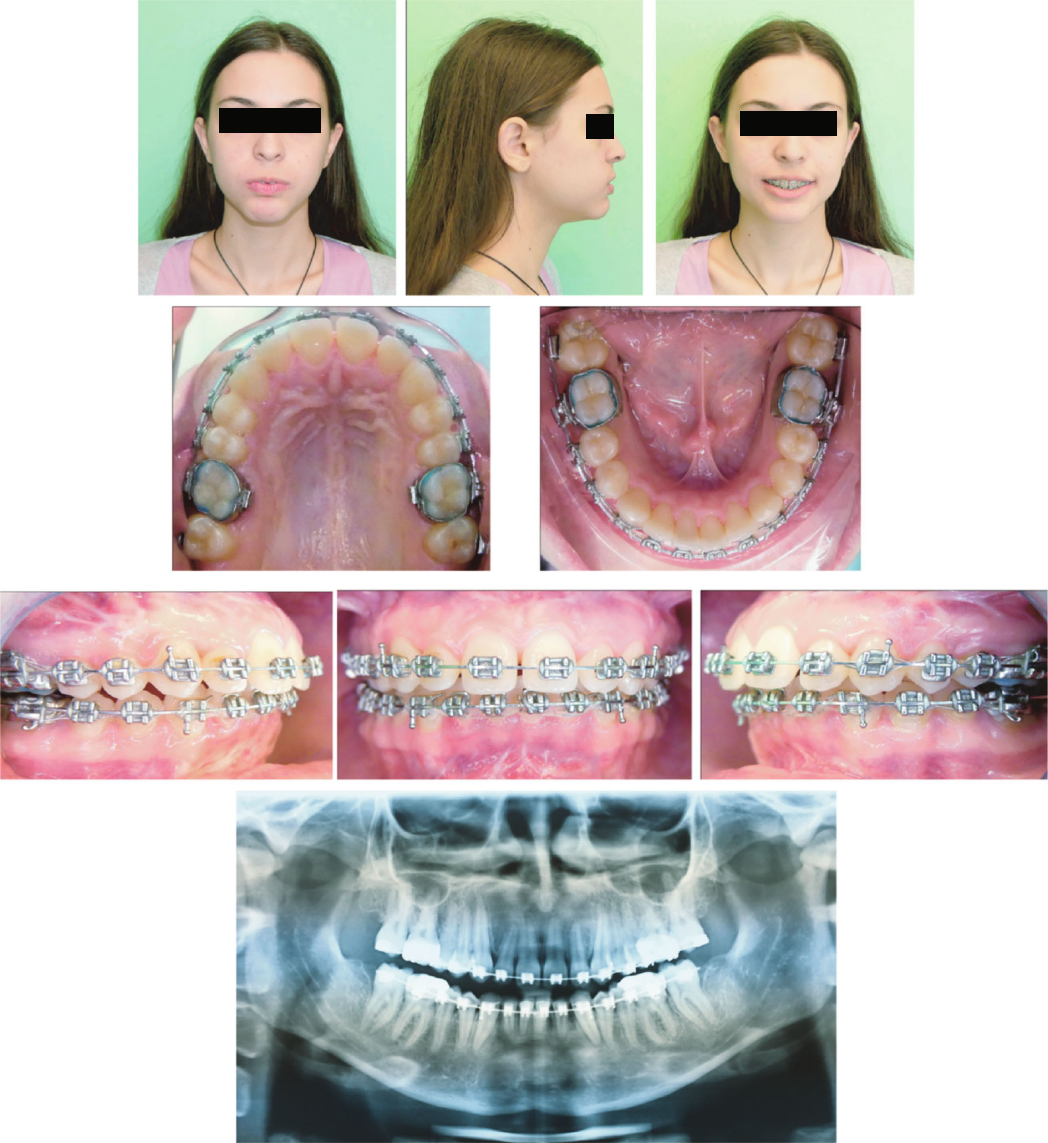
Extraoral photographs (T2), intraoral photographs (T2), and orthopantomogram (T2); T2=treatment progress (18 months).
